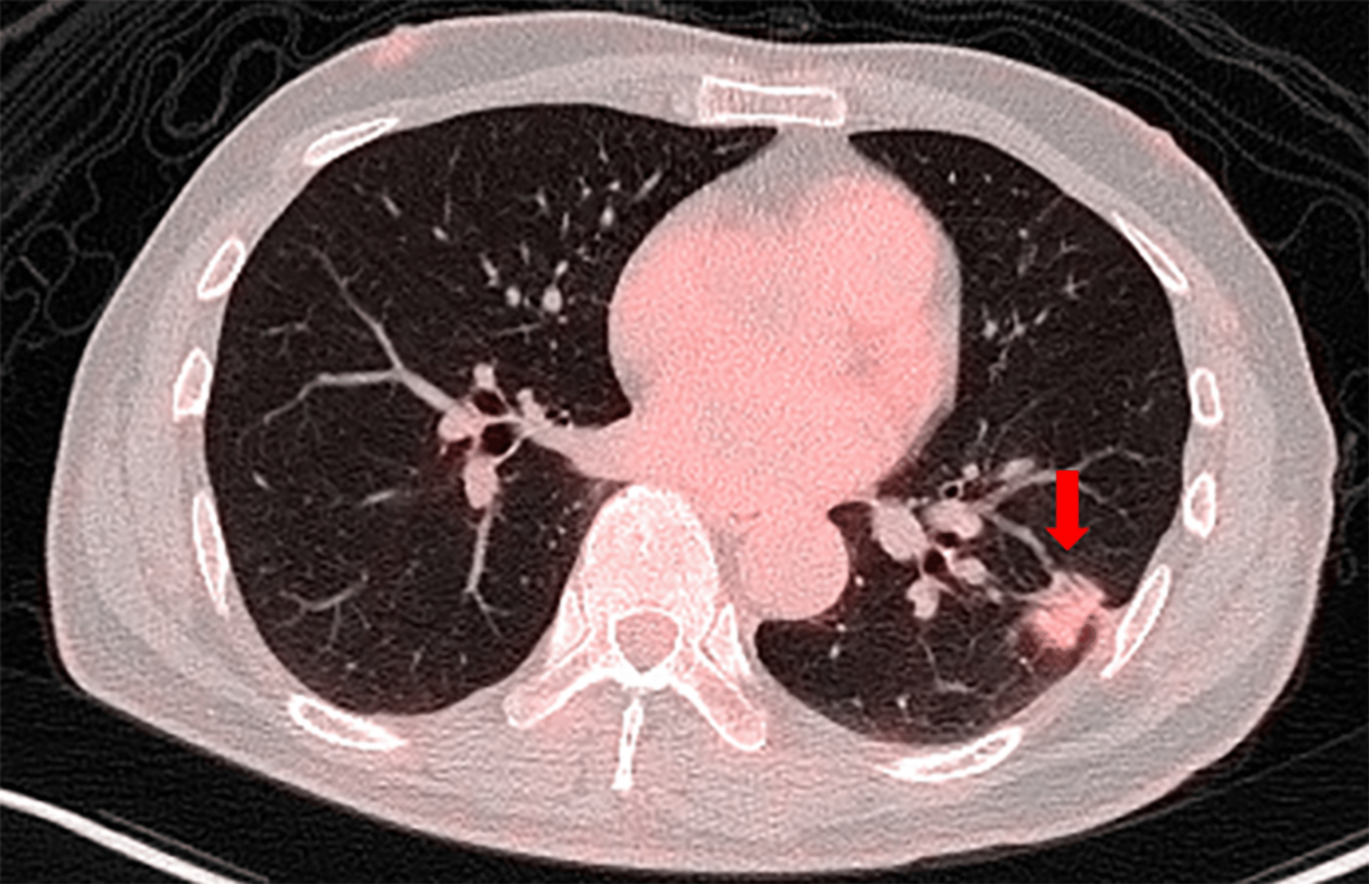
PET-CT findings Fluorodeoxyglucose accumulation was detected in the tumor in the left lower lobe, with an SUVmax of 2.04.
